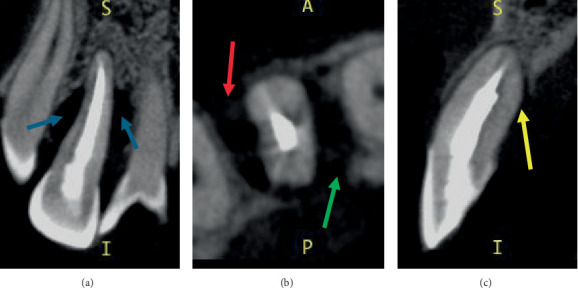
CBCT scan of Tooth 12. (a) Coronal section showing intrabony defects on mesial and distal aspects (blue arrows). (b) Transverse section showing the distal intrabony defect extending to the buccal aspect (red arrow) and the mesial intrabony defect extending to the palatal aspect (green arrow). (c) Sagittal section showing loss of bone on the palatal aspect (yellow arrow).
